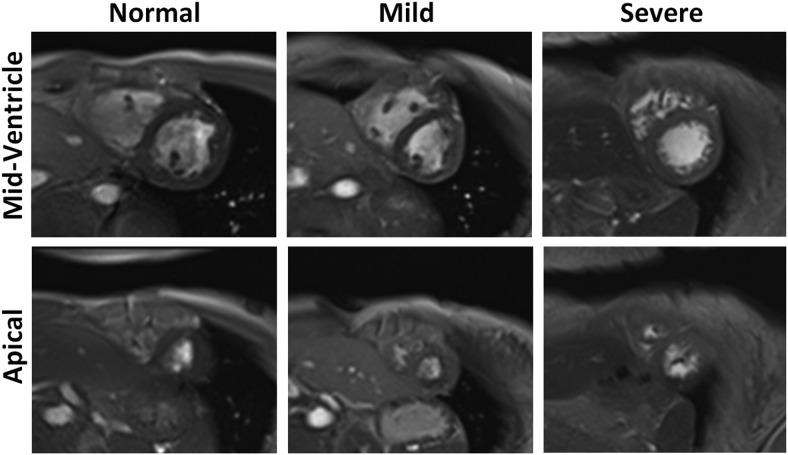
Myocardial trabeculations in the right ventricle, as shown on short-axis cine MR images. Trabeculations are especially apparent at the midventricular (top) and apical (bottom) slices. Examples of normal (left), mild PAH (middle), and severe PAH (right) cases are shown.
